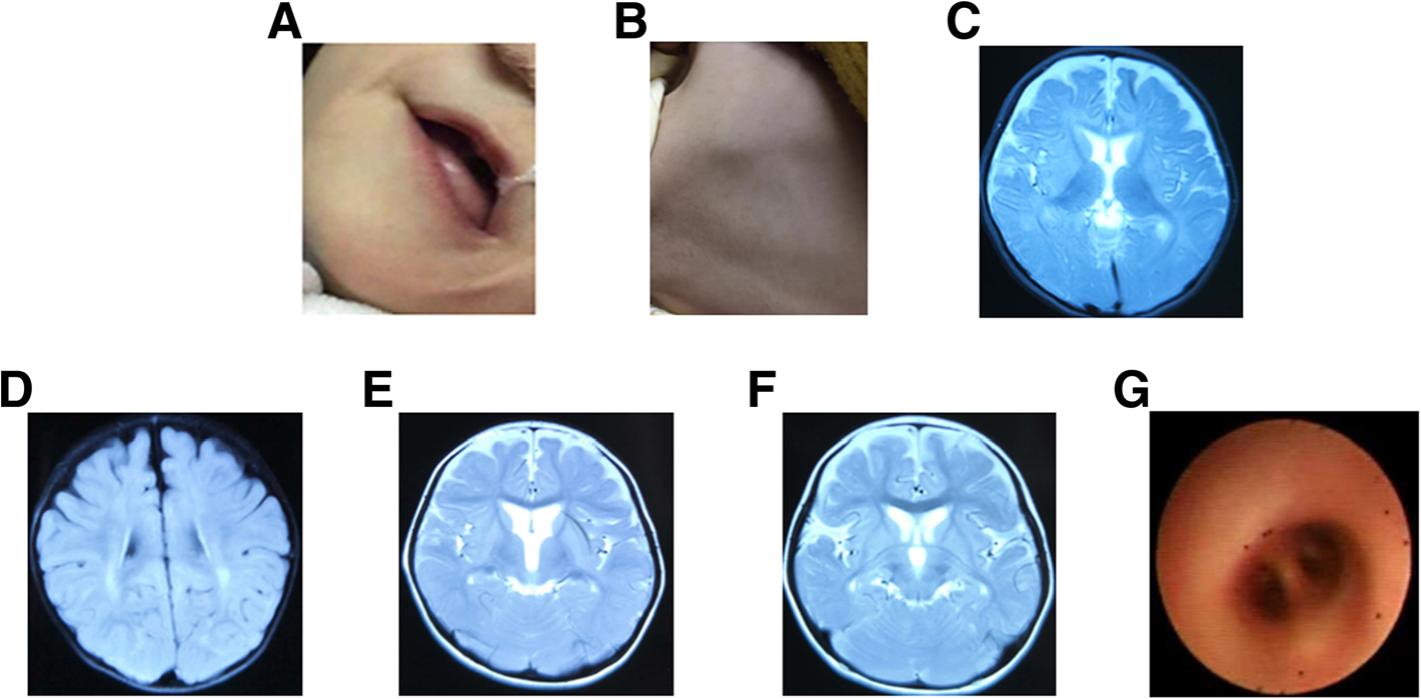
Clinical pictures of this patient. a: Tooth delay or tooth agenesis was found at the age of 2 years and 6 months old; b: Body examination indicated manifestation of polytrichia; c: Brain MRI showed the extra cerebral space widening at six months old; d-f: Frontotemporal space widening, delayed myelination or hypomyelination of white matter in the focal area around the posterior horn of the bilateral lateral ventricles at the age of eleven months. g: Fiberoptic bronchoscopy presented the absence of right middle bronchus orifice
